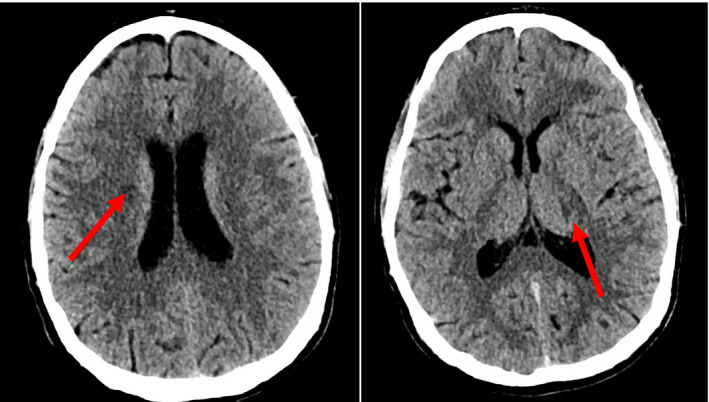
CT Brain without contrast. Obtained during initial workup for the patient showing a poorly defined area of hypoattenuation in the right corona radiata that extends to the lateral margin of the body of the right caudate nucleus. There is a suggestion of a small linear area of hypoattenuation in the lateral aspect of the left side of the thalamus.
